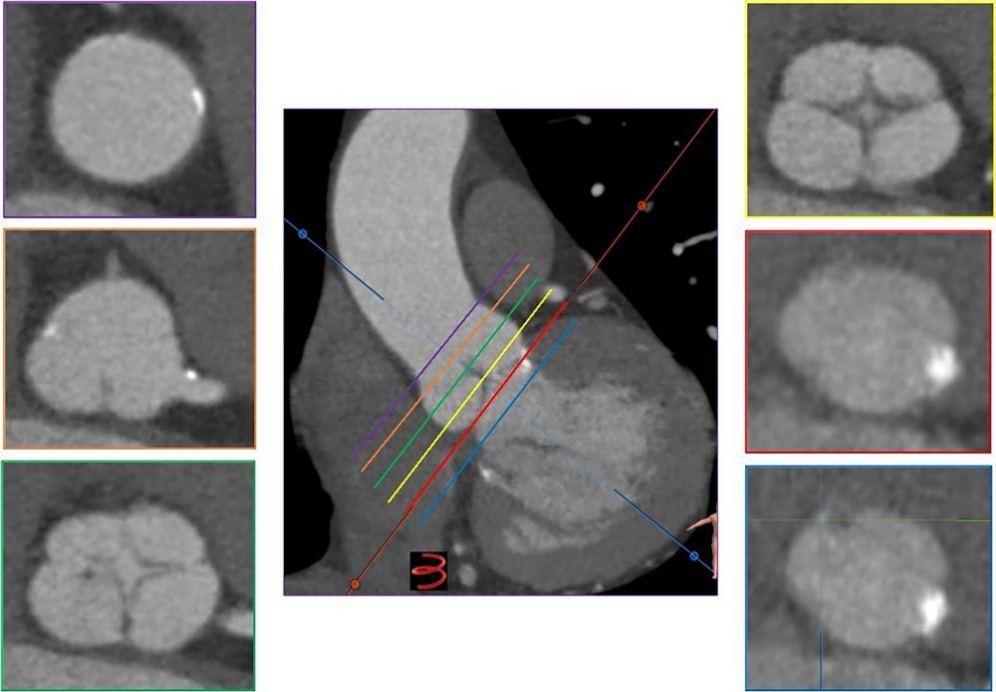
Measurement of virtual landing zone on computed tomography: Descending from the aortic root (purple line), sinutubular junction (orange line), and cusps (green and yellow lines) to the left ventricular outflow track (line blue), the hinge point of each individual aortic valve cusp is identified to establish the plane of the annulus (red line). Virtual ring of aortic annulus was selected at the plane of the circumferential ring at the basal attachment points of the aortic cusps (red line).
